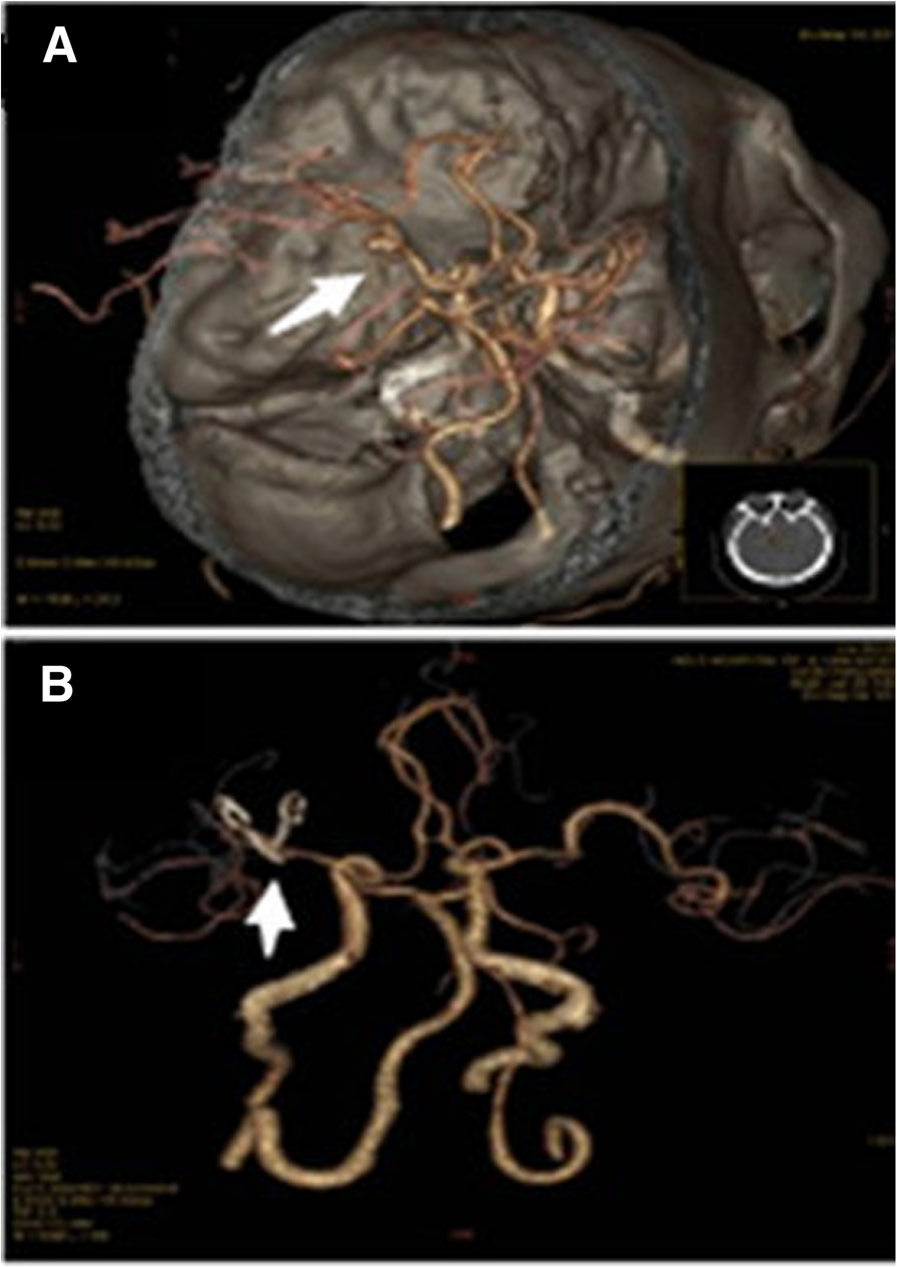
a CTA demonstrated that there was vesicle-like bulges in the left middle artery bifurcation and the formation of asci on the vesicle was visible. b Head CTA revealed that vessels in the middle cerebral artery bifurcation were in good shape, and no residual aneurysm neck and relapse were observed
